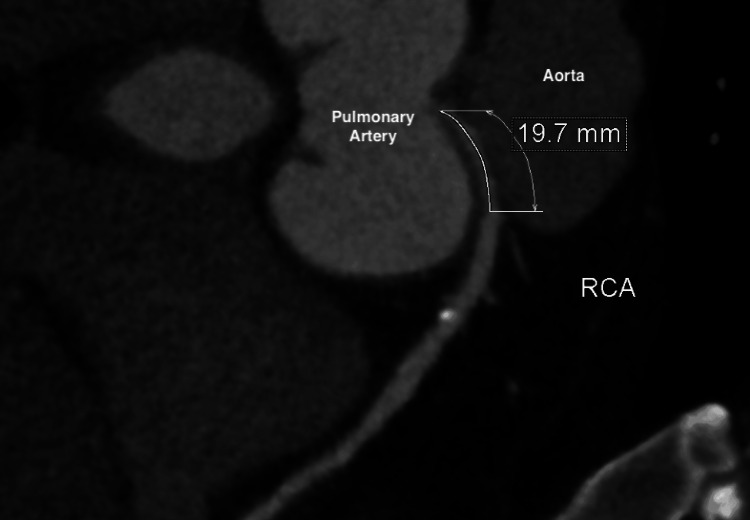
Coronary computed tomography angiography of anomalous right coronary artery Anomalous right coronary artery (ARCA) originating from the opposite sinus with an interarterial course traveling between the ascending aorta and the pulmonary artery. The proximal segment measures approximately 19.7 mm.
